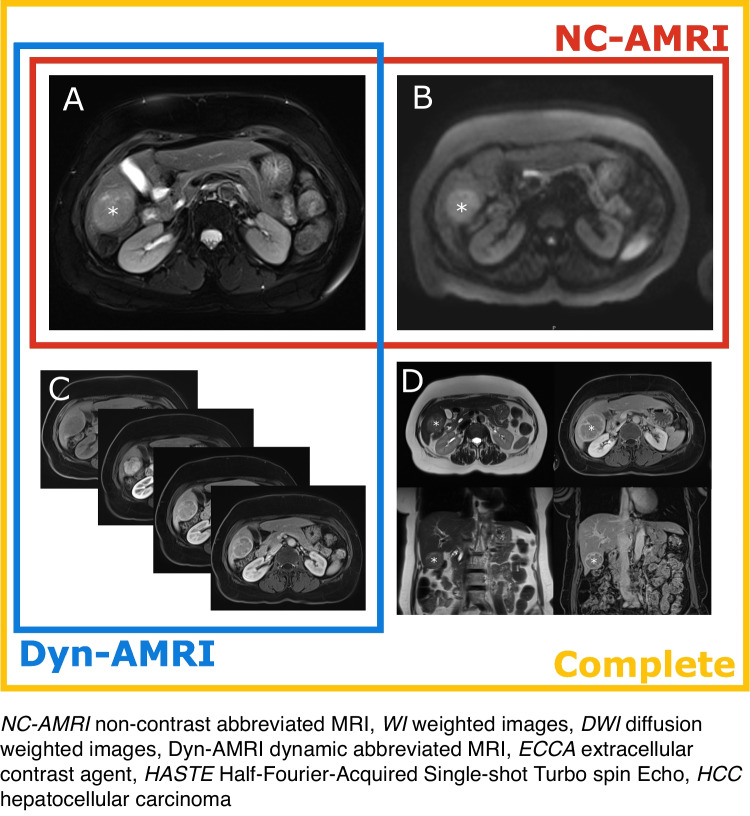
Sequences included in our two AMRI protocols (NC-AMRI, Dyn-AMRI) and complete MRI. NC-AMRI (red square) included fat-saturated T2WI (A) and DWI (B). Dyn-AMRI (blue square) included fat-saturated T2WI (A), native T1WI and dynamic ECCA-enhanced T1WI arterial, portal and transitional phase (C), without delayed axial and coronal 5 min phase. Complete MRI (yellow square) included fat-saturated T2WI (A), axial and coronal T2WI HASTE (D), DWI (B), native T1WI and dynamic ECCA-enhanced T1WI arterial, portal, transitional (C) and delayed axial and coronal 5 min phase (D). To note, a LI-RADS 5 biopsy-proven HCC of segment VI is visible on all sequences (asterisks)
